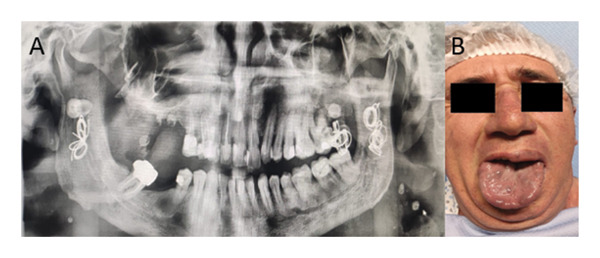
Complex airway involvement in a patient with SWS and OHS with oral and tongue angiomatosis. (A) Panoramic radiograph showing the outcomes of interventional radiologic embolization for oral cavity angiomas following spontaneous hemorrhage, which necessitated an emergency tracheostomy due to a difficult airway. (B) Clinical image depicting multiple vascular angiomas on the posterior surface of the tongue, contributing to significant airway compromise and posing major challenges for intubation.
